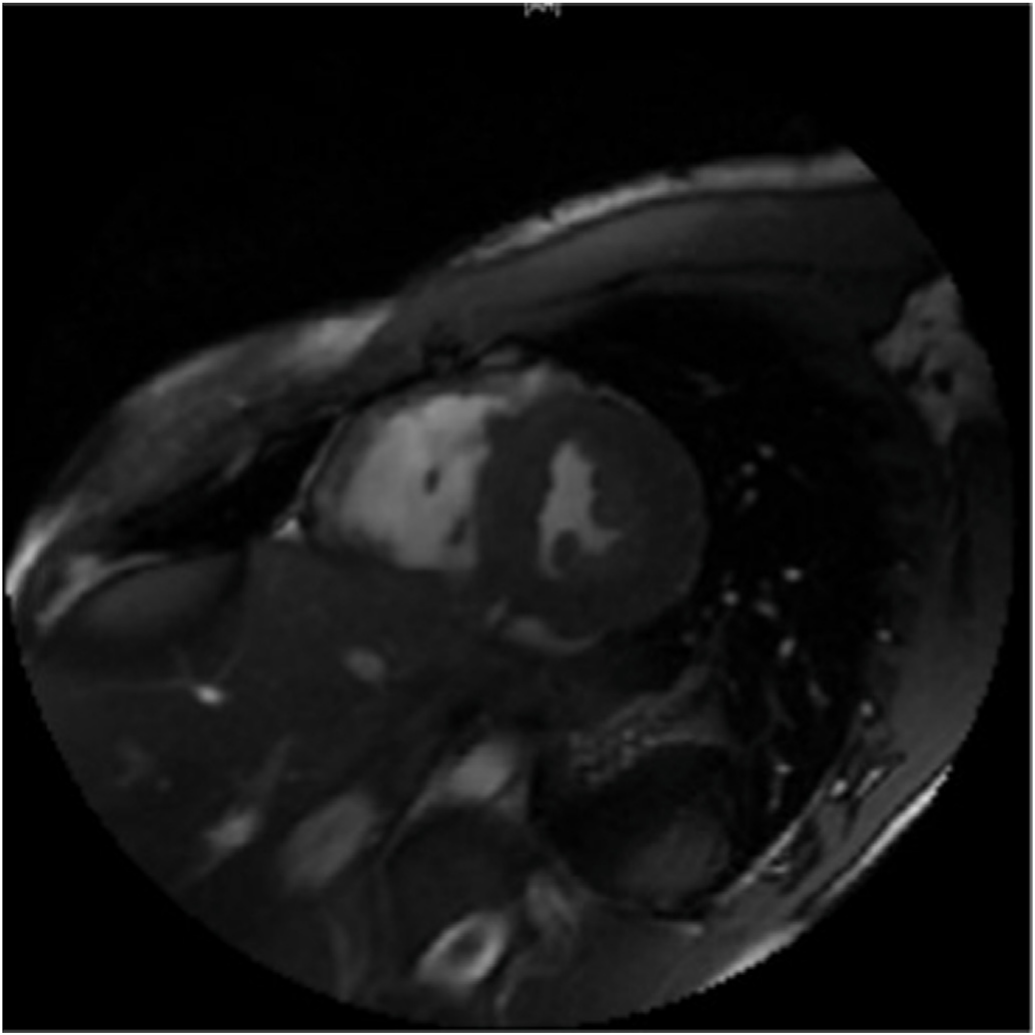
Transverse T2 gated MRI image depicting moderate right ventricular dilation with segmental wall thinning. In contrast the LV is normal in size with normal wall thickness.
